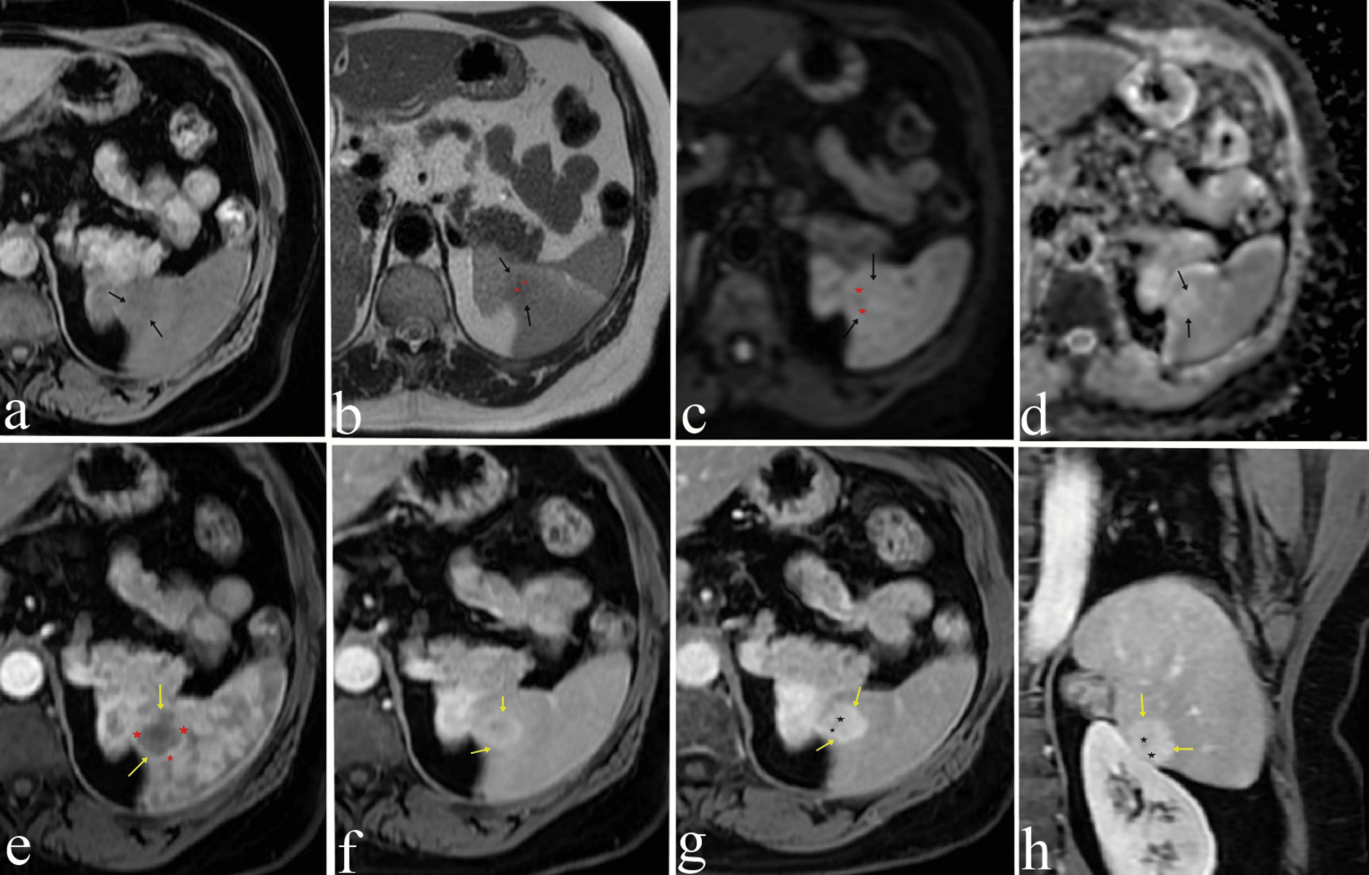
Dynamic MRI examination On non-contrast fat-suppressed T1-weighted axial imaging (a) and T2-weighted axial imaging (b), the lesion (black arrows) demonstrates iso-hypointense signal characteristics. On diffusion-weighted imaging (c) and ADC imaging (d), no significant diffusion restriction is observed. In T2-weighted imaging (b) and diffusion-weighted imaging (c), a centrally located hypointense scar (red asterisks) is noted. Following the administration of contrast material, on arterial T1-weighted imaging (e), the lesion (yellow arrows) demonstrates weak peripheral enhancement (red asterisks) compared to the splenic parenchyma. On post-contrast portal venous phase (f), delayed venous phase axial (g), and coronal (h) images, the lesion exhibits progressive centripetal enhancement (yellow arrows). In delayed venous phase images (g, h), hypointense signal characteristics consistent with a central scar are observed (black arrows).
